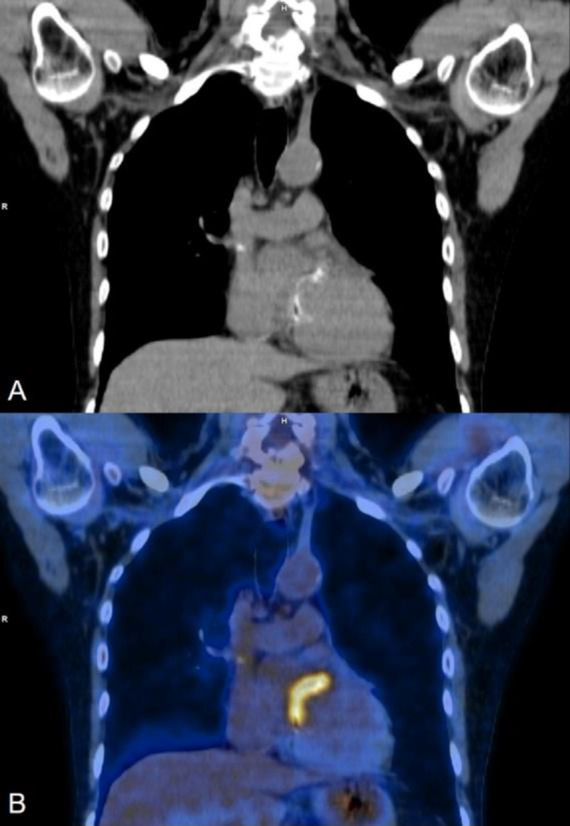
Coronal section (A) CT and (B) fused FDG PET CT images showing intense FDG uptake (SUV_Max_: 6.1) along the prosthetic mitral valve
